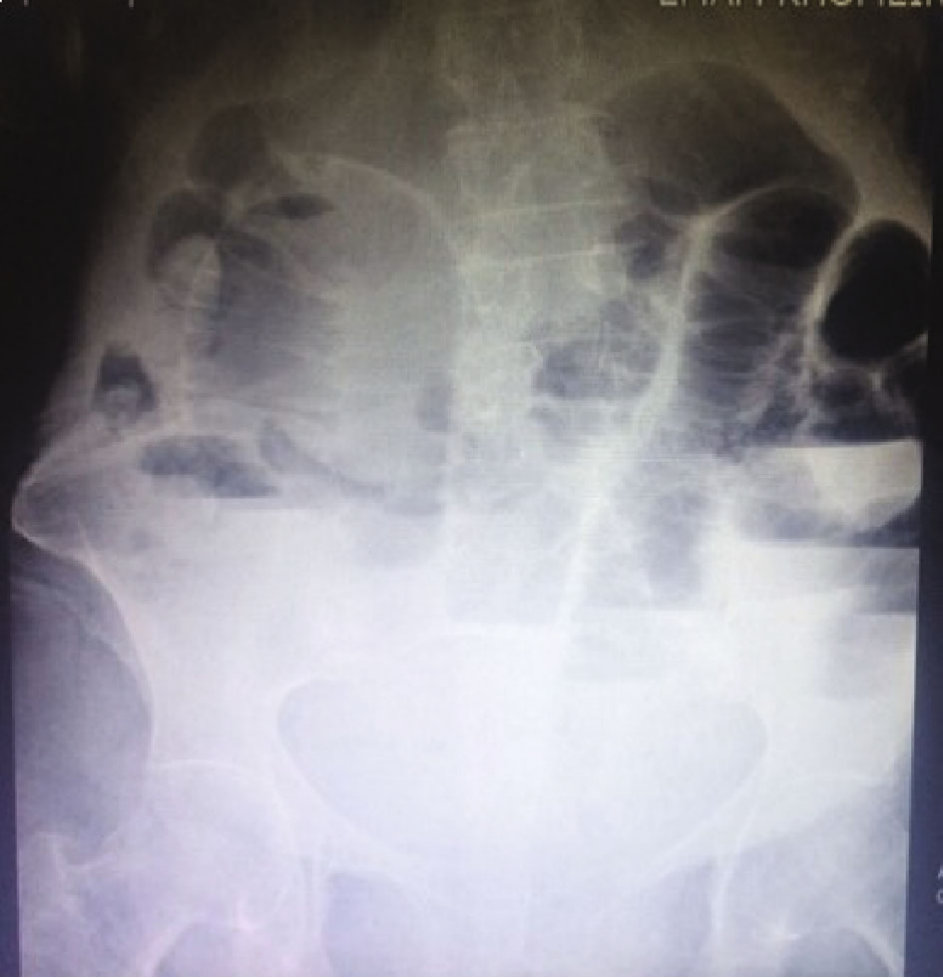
Upright abdominal X-ray.
